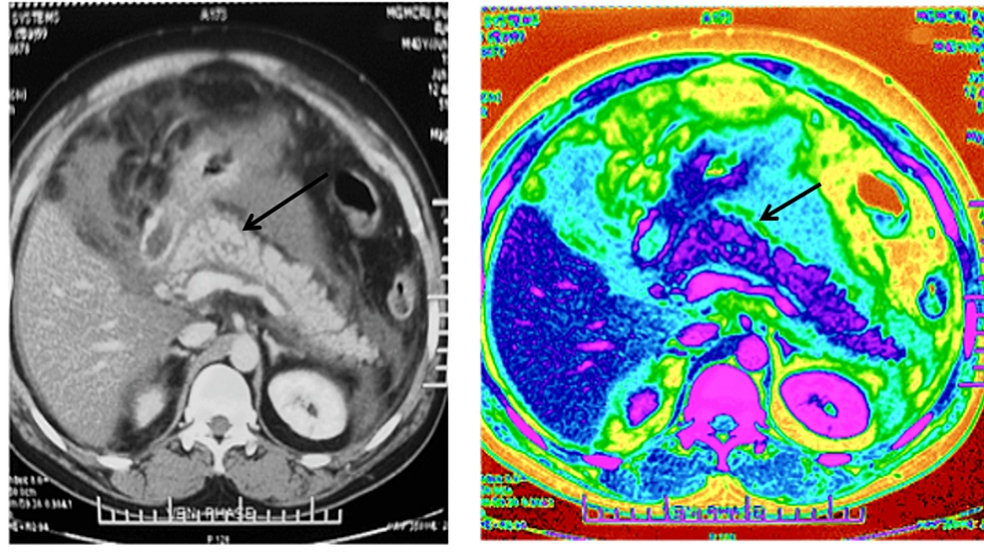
A: Contrast-enhanced computed tomography (CECT) image of patient in Case 1 showing normal enhancement of entire pancreas with preserved lobularity of the gland (black arrow). B: In the processed image of patient in Case 1, preserved layer of anterior pancreatic capsule is depicted in green colour (black arrow) highlighting the intactness of pancreas in extrapancreatic necrotising pancreatitis.
